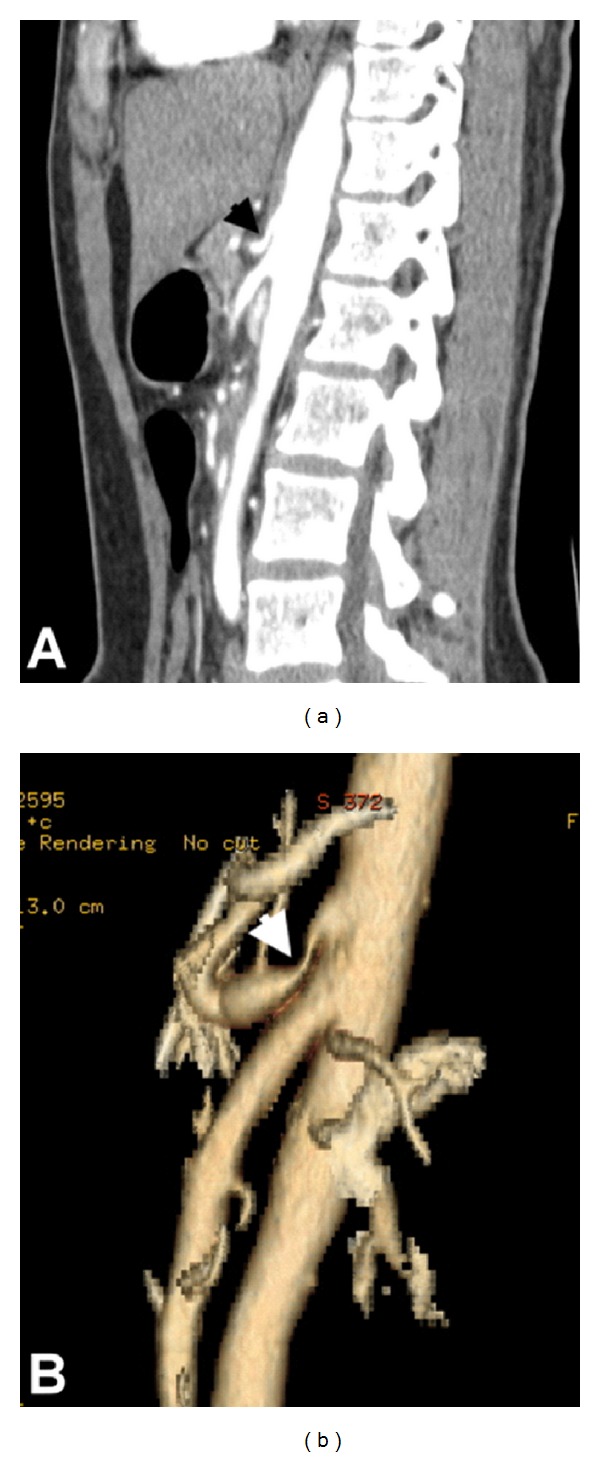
Computed tomography angiography of the celiac artery compression syndrome demonstrated the characteristic hooked narrowing of the proximal celiac artery with poststenotic dilatation in 2D reconstruction image (a, arrow) and 3D reconstruction image (b, arrow).
